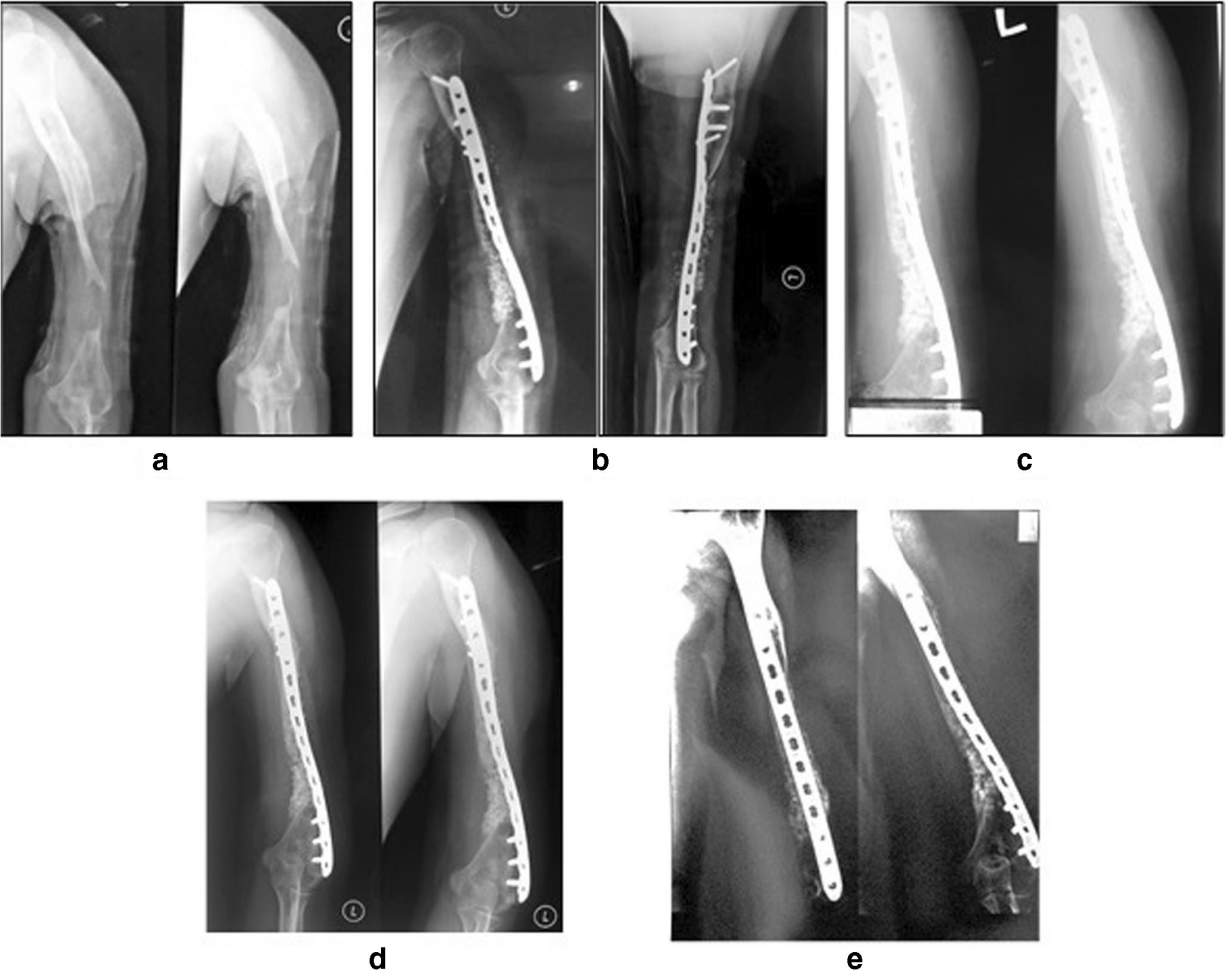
An 18-year-old male (case 1) with 5-cm bone defect of the humerus. a Pre-operative radiography. b Post-operative radiography. c Two-month post-operative radiography. d Six-month post-operative radiography. e Twelve-month post-operative radiography
